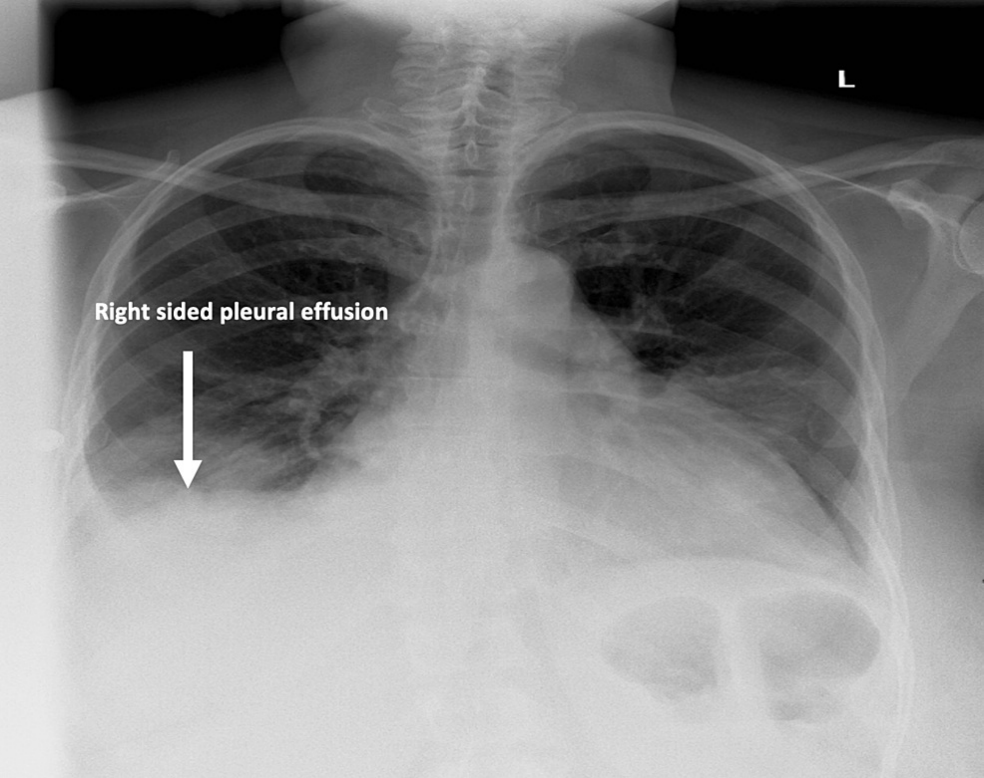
Chest X-ray showing bilateral pleural effusions more pronounced on the right (arrow).
